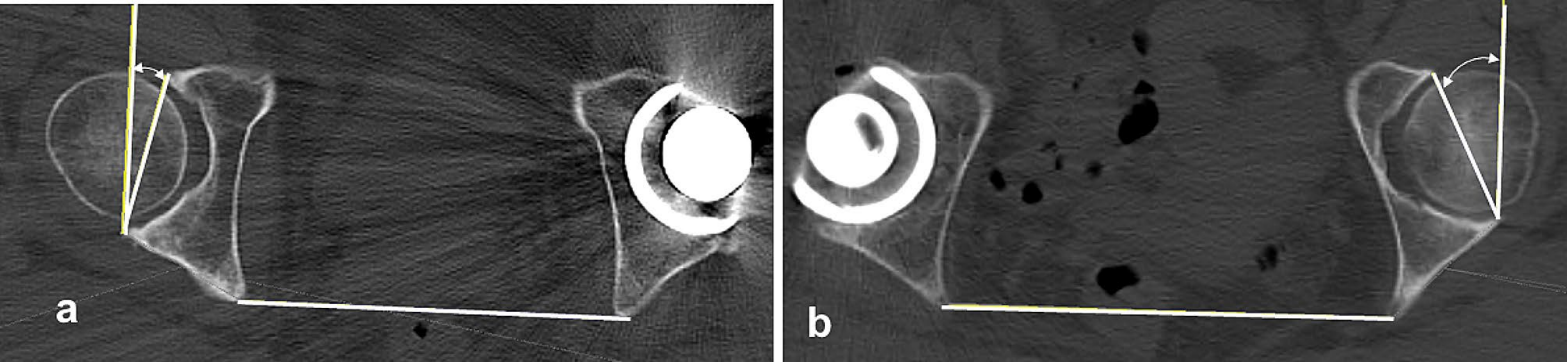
Measurement of the acetabular anteversion angle. The CT axial image at the hip center was selected. The acetabular anteversion angle (white curved double arrow) was defined as the angle between a reference line perpendicular to a line connecting the posterior ischia and a line connecting the posterior and anterior margins of the acetabulum. a A coxa profunda-negative case. b A coxa profunda-positive case. CT, computed tomography
